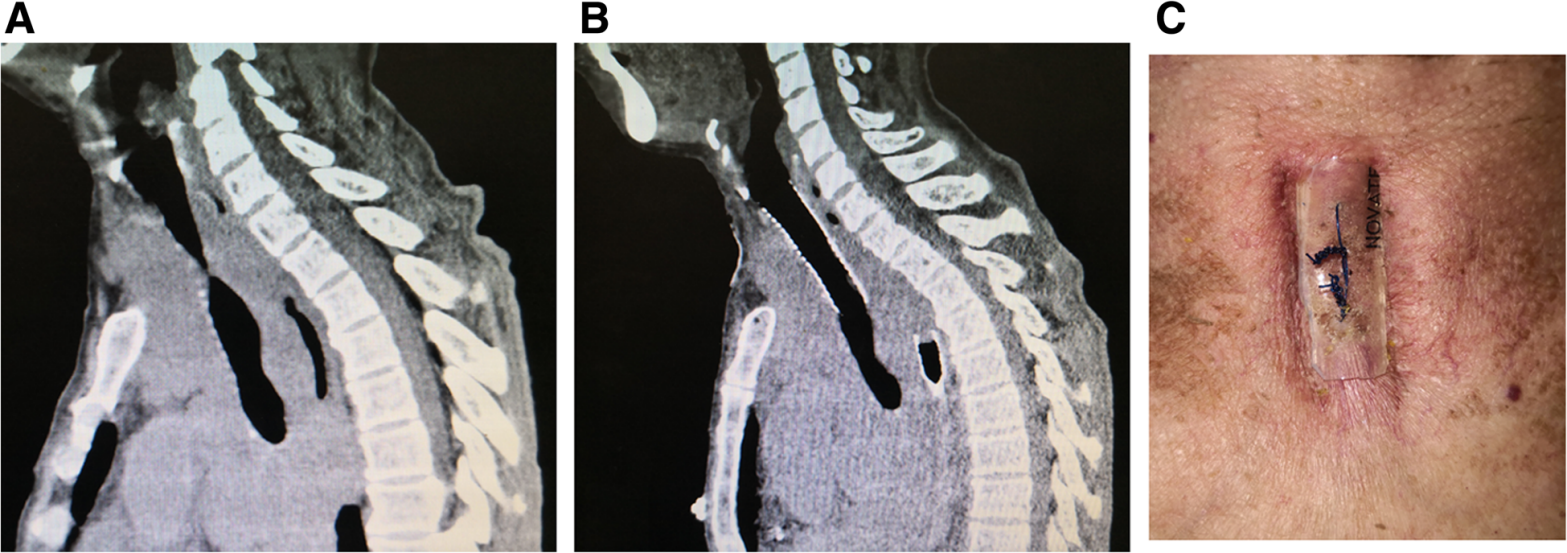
Preoperative and postoperative images of one patient (Case No. 9). a: Sagittal preoperative CT showing the upper tracheal stenosis. b: Postoperative sagittal CT showing the normal trachea. c: The appearance of the suture on the neck
